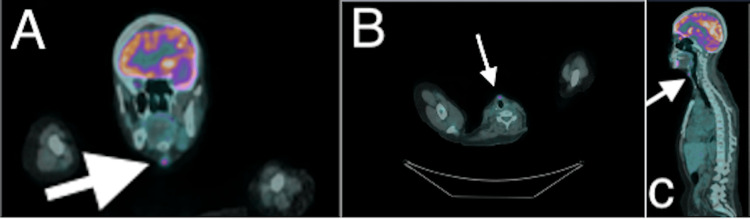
PET scan post resection and rituximab, cyclophosphamide, doxorubicin, vincristine, and prednisone (R-CHOP) (A) Transverse horizontal, (B) coronal, and C) sagittal section midline FDG-avid lesion measuring 1.2 × 0.8 cm anterior to the cricothyroid cartilage initially mistaken for recurrence. istopathological assessment revealed a benign nature.
